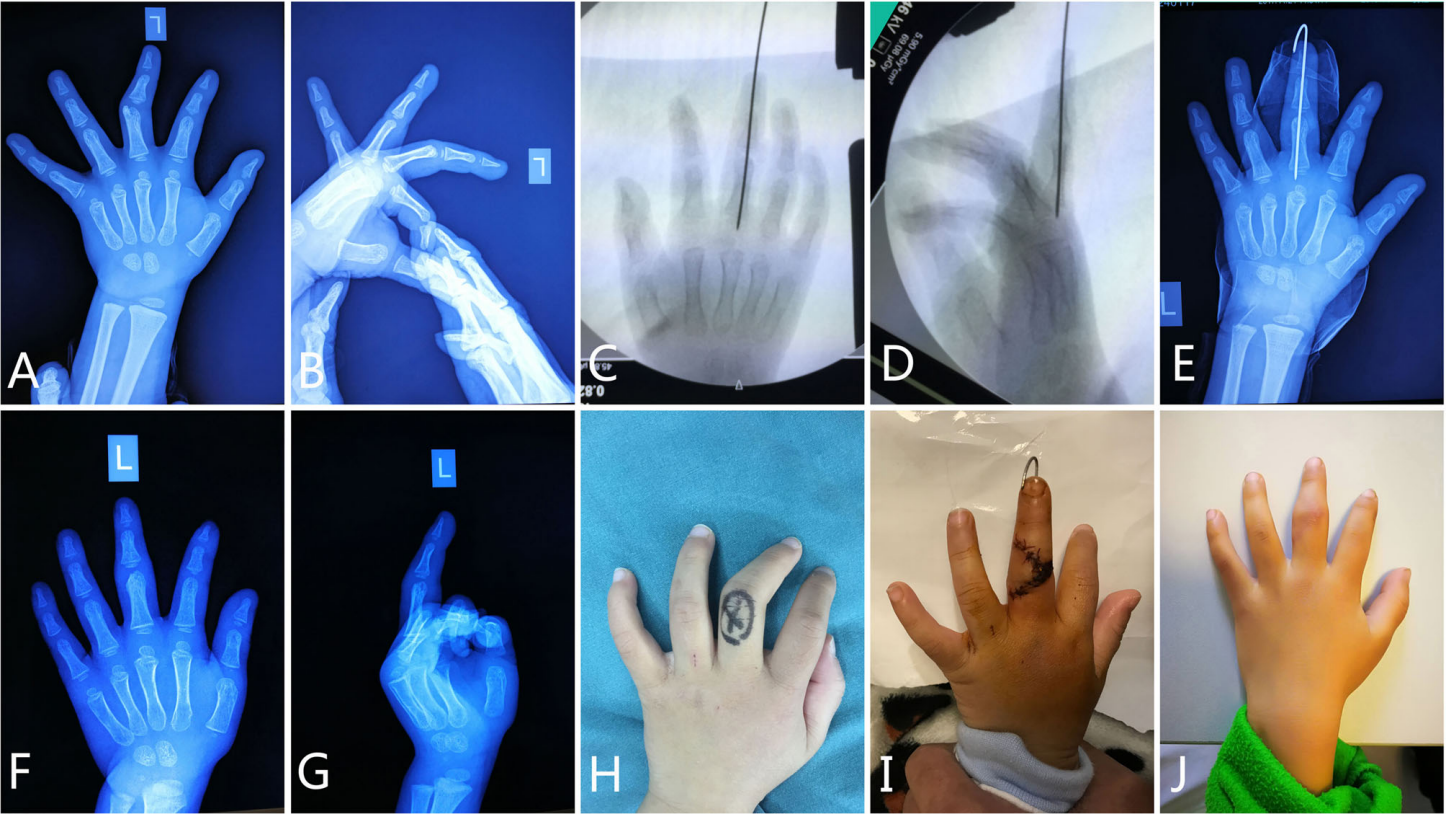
Diagnosis and treatment of clinodactyly deformity in a 2-year-10-month-old boy. The radiograph on presentation showed an outgrowth around the distal end of the third proximal phalanx (A, B). During the surgery, the abnormal mass was resected and a K-wire was implanted to keep the finger straight (C, D). After operation, the radiographs showed good prognosis (E–G). Clinical examination established the diagnosis of clinodactyly deformity of his third finger (H), and also showed essentially normal movement of the upper extremity at the follow-up (I, J).
